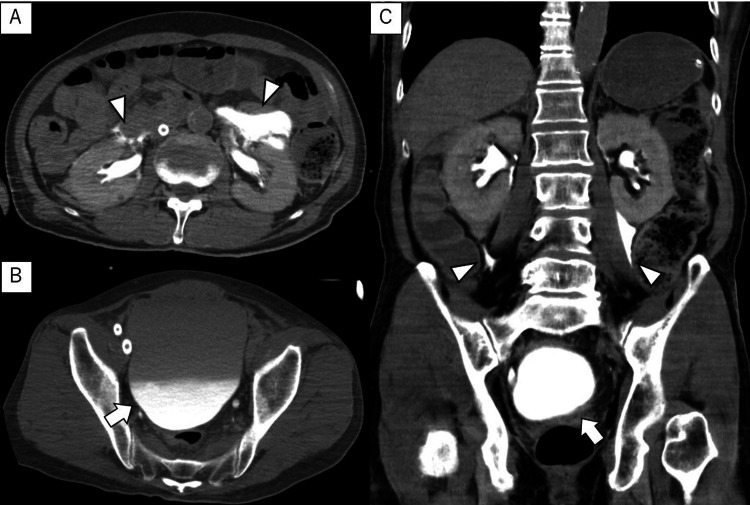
CT imaging before transferring to the ICU Axial and coronal contrast-enhanced CT imaging before the patient was transferred to the ICU showed the leakage of the contrast medium used during CAG in the bilateral renal pelvis and perirenal region (white arrowhead), along with urinary retention (white arrow) CT: computed tomography; ICU: intensive care unit; CAG: coronary angiography
